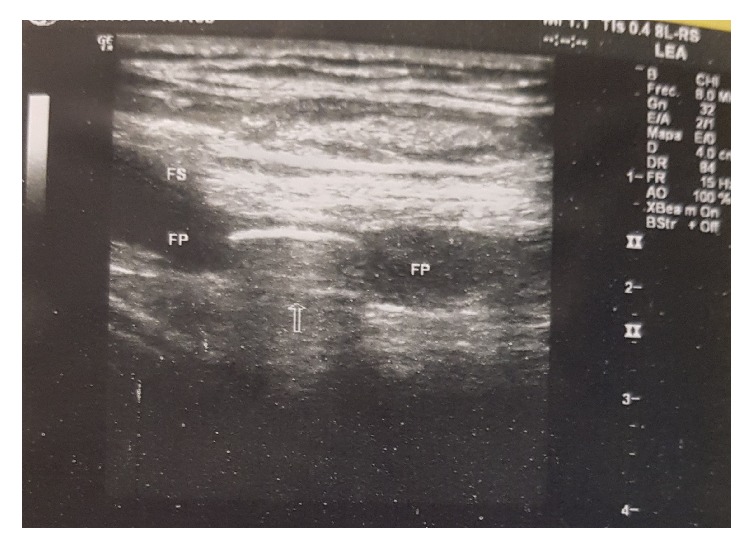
Lower right limb ultrasound shows the bullet at division of femoris artery, with obstruction of profundal femoris artery.
